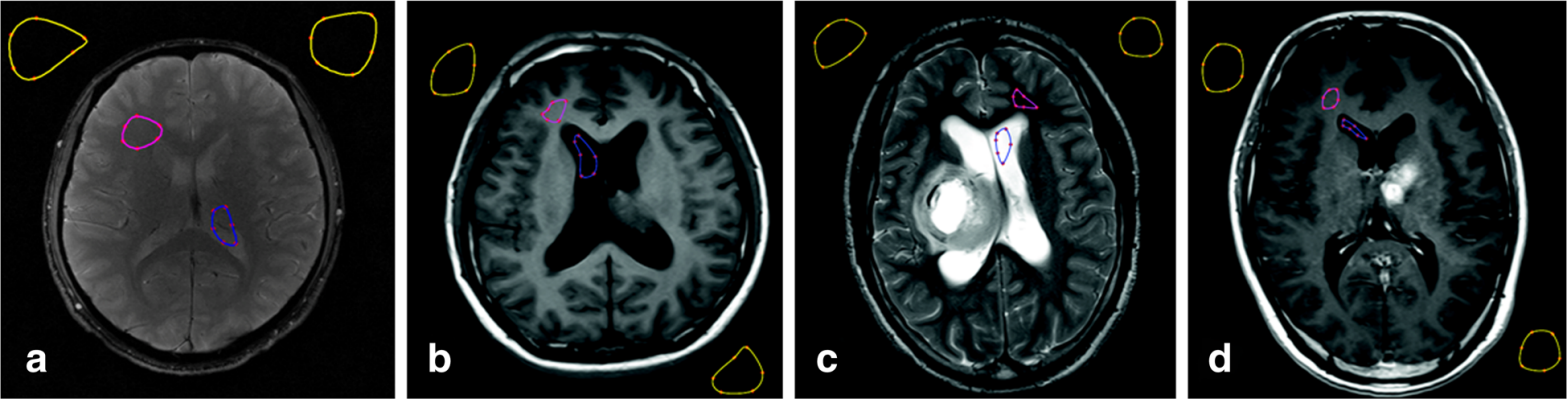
Manual outline of tissue regions and air regions. a, b, c, d are T_2_^*^, T_1_, T_2_ and T_1_C weighted MR images, respectively. b, c, d demonstrates one example of a subject. Primarily points localized by observers are noted with red sparkles. Outlined WM, CSF and AIR regions are in closed curves with pink, blue and yellow lines, respectively. Note that images have been cropped for display purpose
